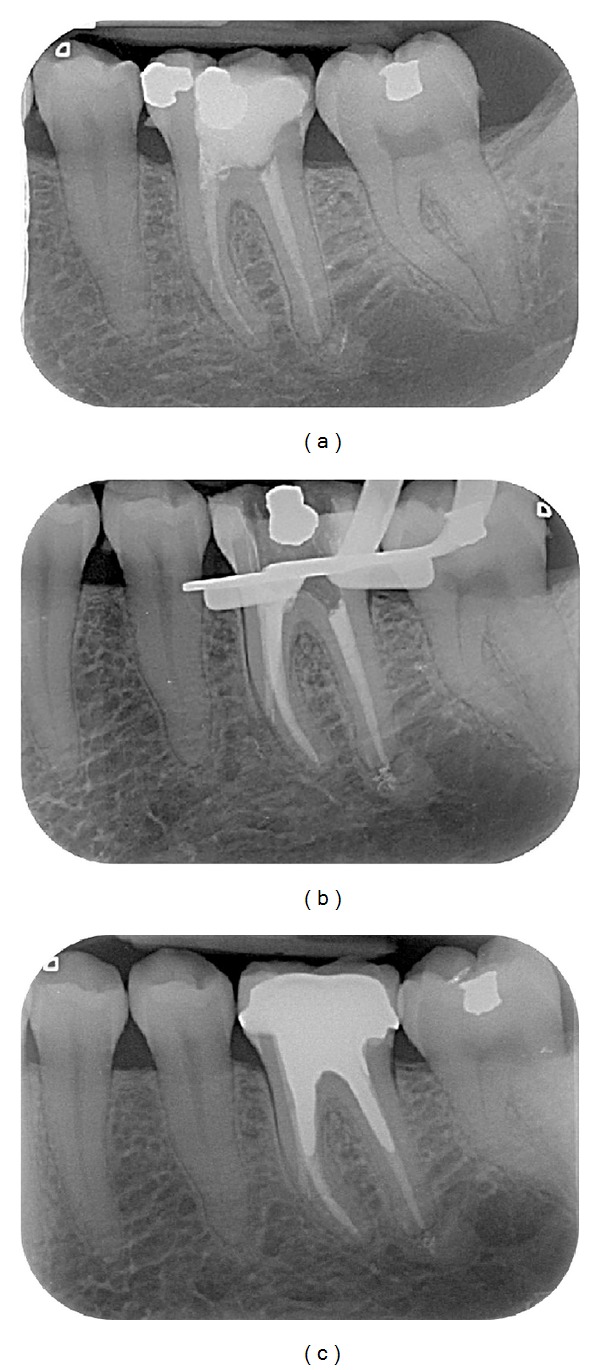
Successful endodontic retreatment of 36 symptomatic, with root perforation. (a) Preoperative X-ray: note the lateral periodontitis regarding the mesial perforation of the mesial root. (b) Peroperative X-ray: the perforation was filled with MTA and the root canal by vertical condensation of warm gutta-percha. (c) Follow-up for 1 year: tooth classified as “healed” (no symptoms, no radiolucency).
